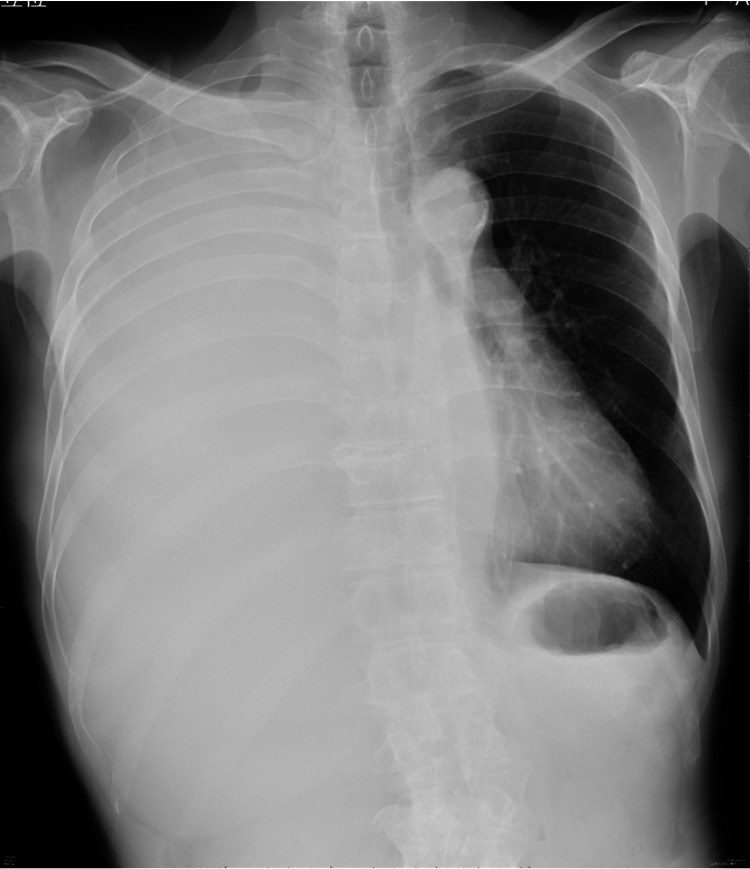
Chest X-ray at the first visit to our hospital Chest X-ray at the first visit to our hospital showed large amount of pleural effusion.
